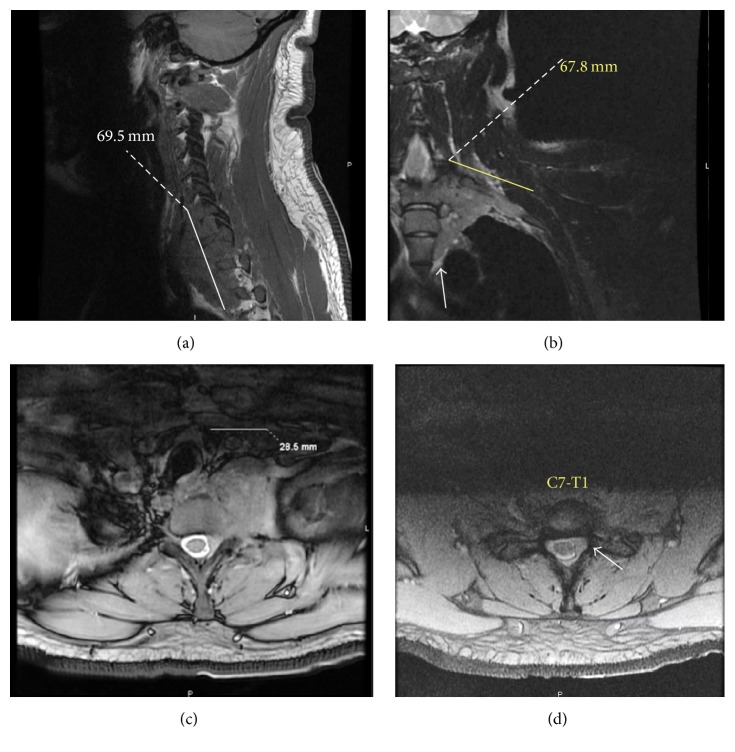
Magnetic Resonance Imaging (MRI) of the cervical spine and brachial plexus at cervical-thoracic levels: T2-weighted images with left paraspinal soft tissue mass and brachial plexus infiltration in sagittal (a), coronal (b), and axial (c) views and intraspinal extension (d).
